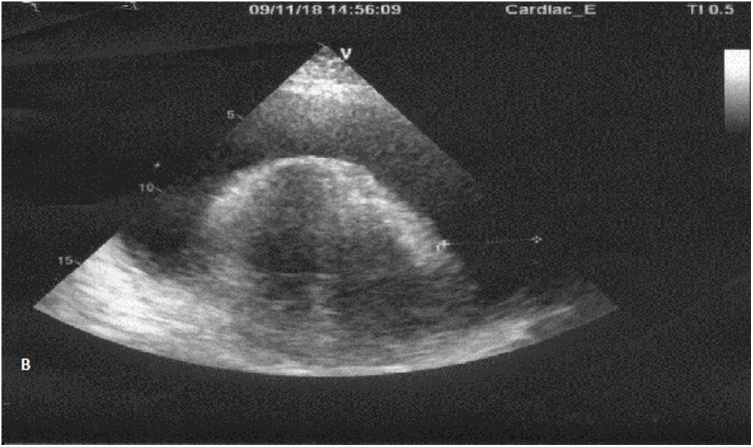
Echocardiography image of a massive pericardial effusion.
